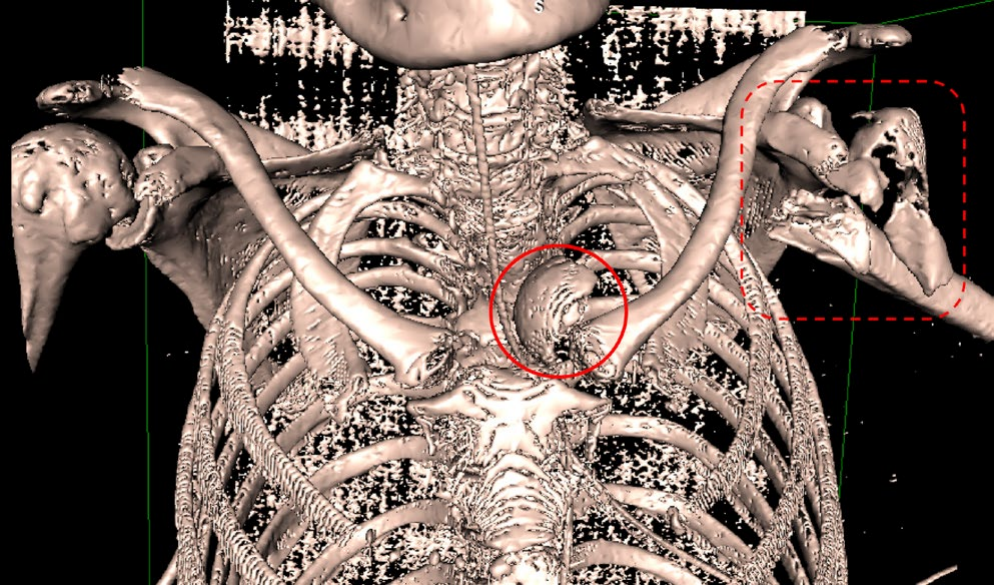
A suggestive three‐dimensional surface rendering image from a chest CT scan shows the dislocation of the HH into the mediastinum (red circle) and the HH fracture (dotted red box).
